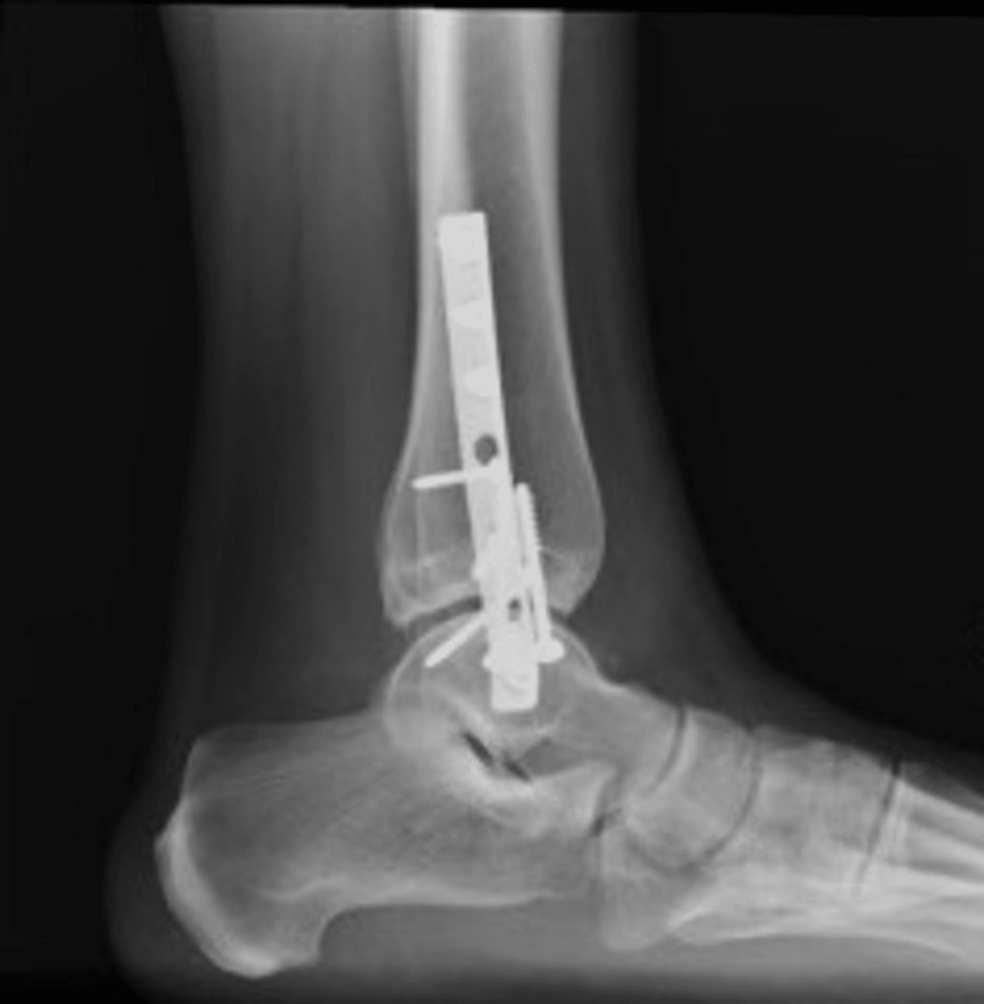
Lateral X-ray view of the bi-malleolar fracture treated with plate and screws showing good bony healing
